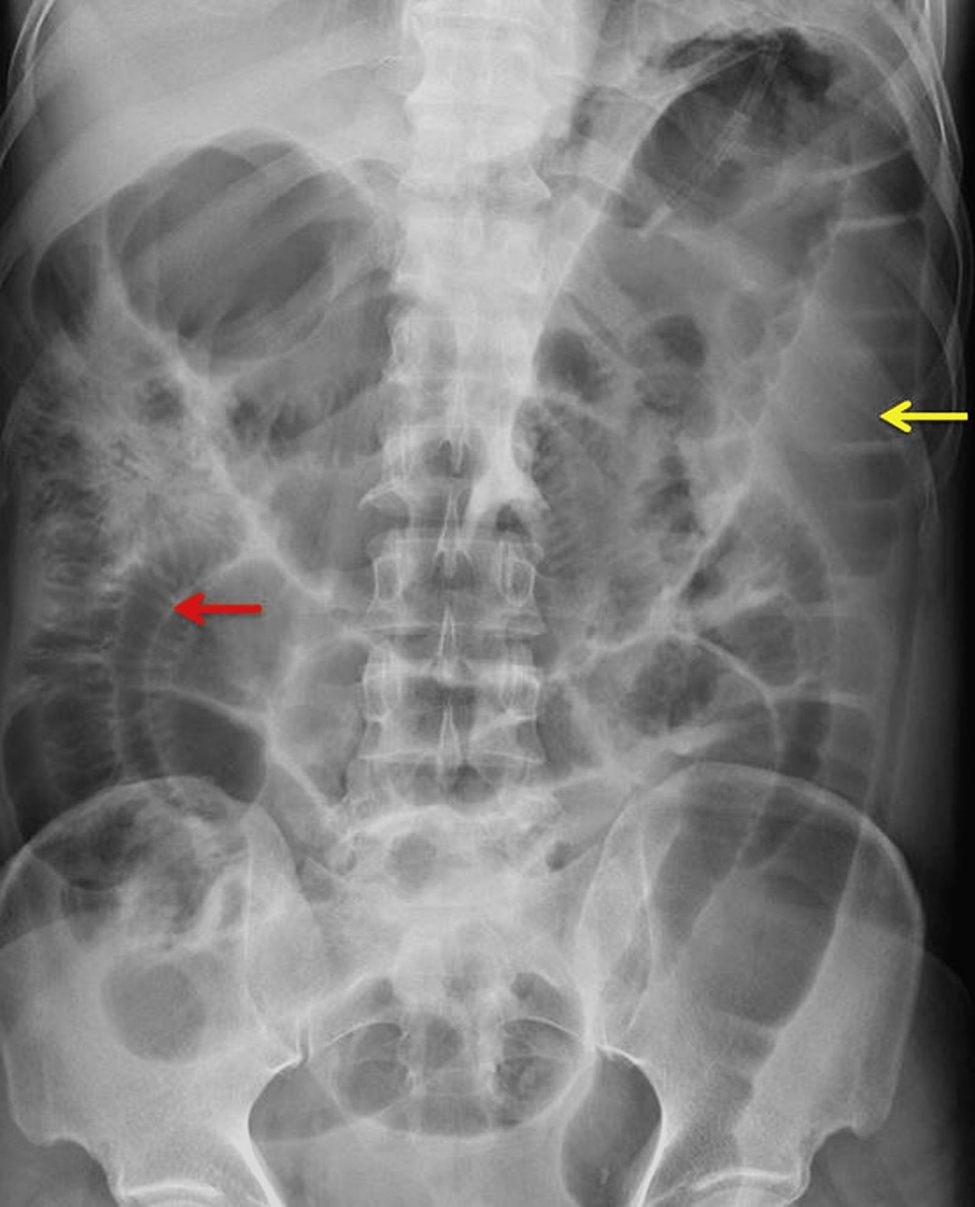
X-ray of the abdomen showing a dilated large bowel loop (yellow arrow) and small bowel loops (red arrow).
